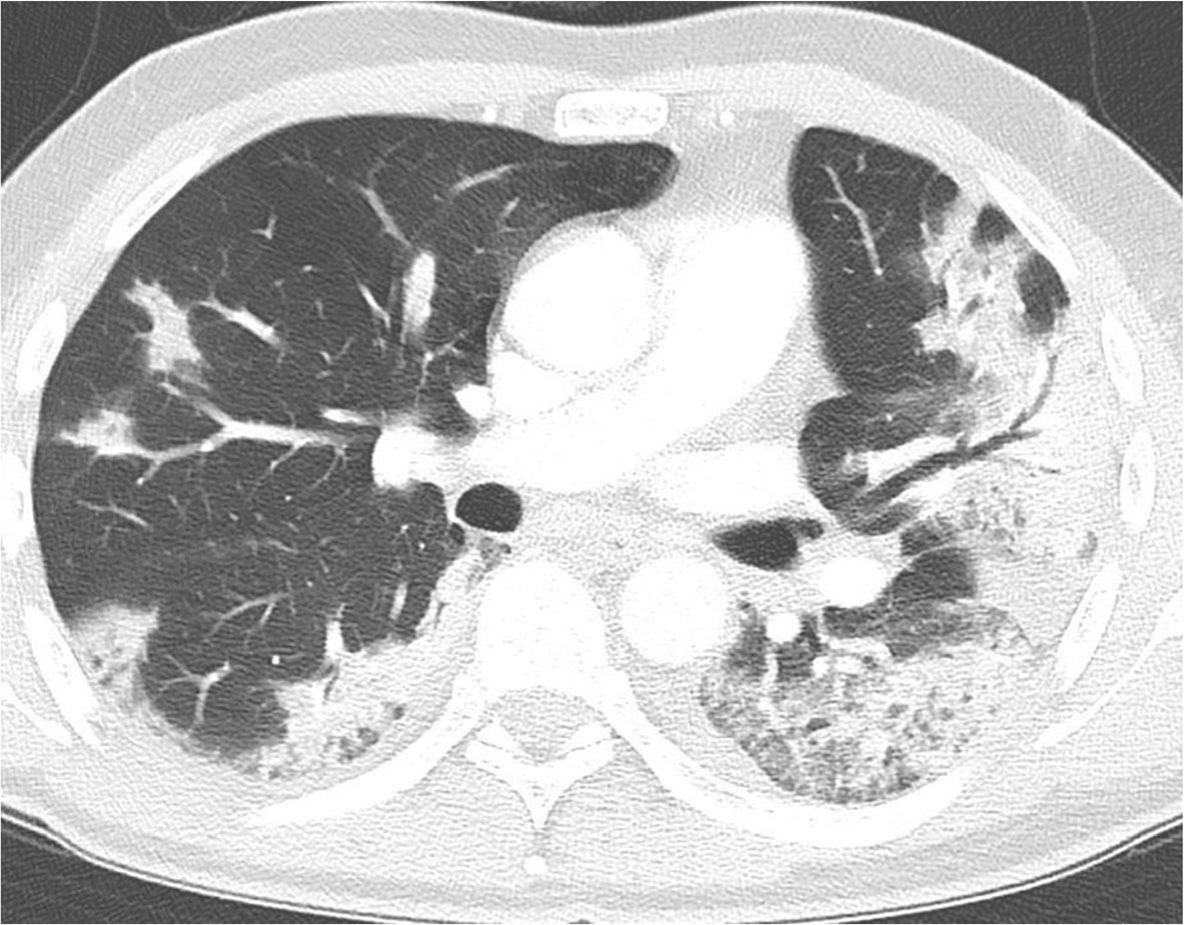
Chest CT showing bilateral COVID-19 changes
